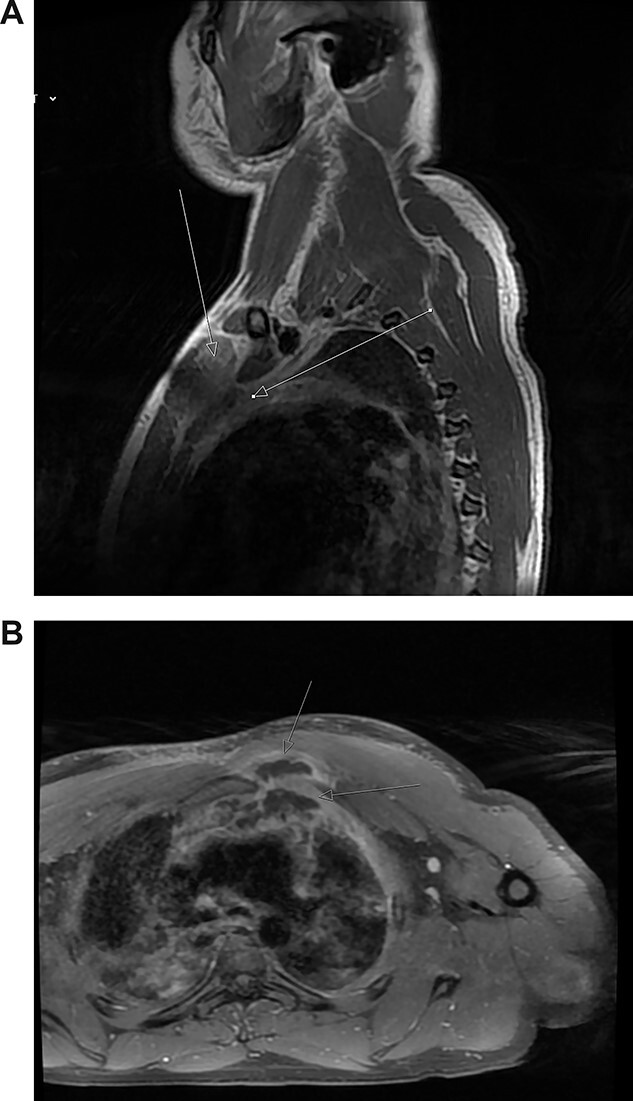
(A) Post-contrast MRI scan showing areas enhancement around pectoralis major (myositis) with extension into the mediastinum (mediastinitis) (*white arrows*). (B) New rim-enhancing collections within left pectoralis major muscle. The collection appears to extend posteriorly into the anterosuperior mediastinum (*white arrows*)
